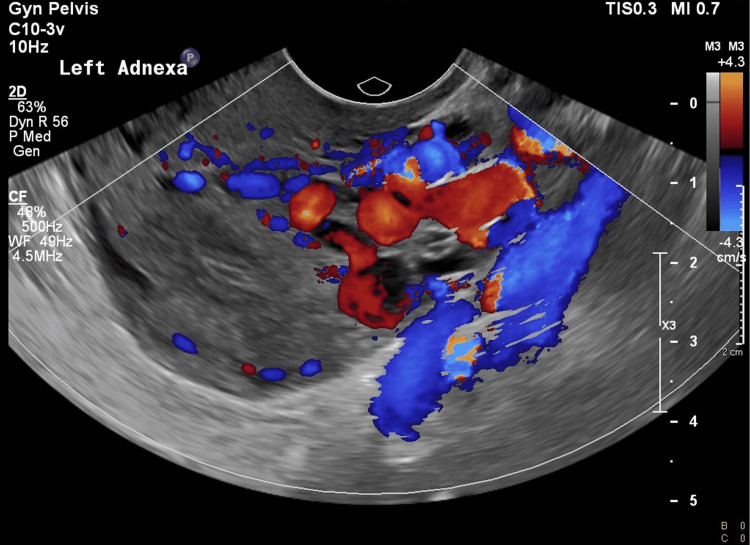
Pelvic ultrasound with color Doppler demonstrates dilated left adnexal veins
